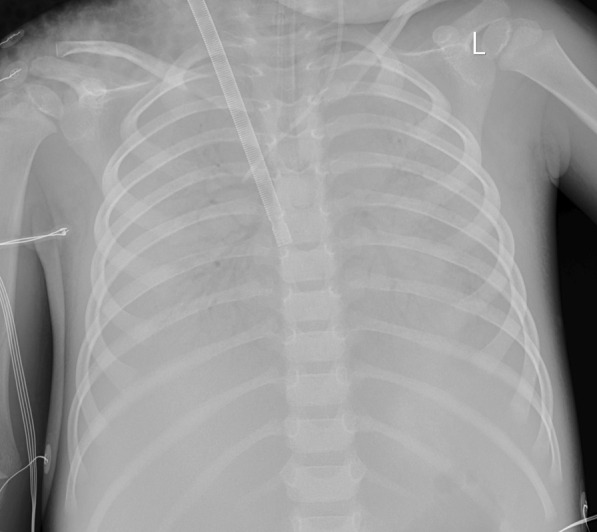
Chest X-ray during extracorporeal membrane oxygenation treatment diffuse density enhancement shadow in both lungs
